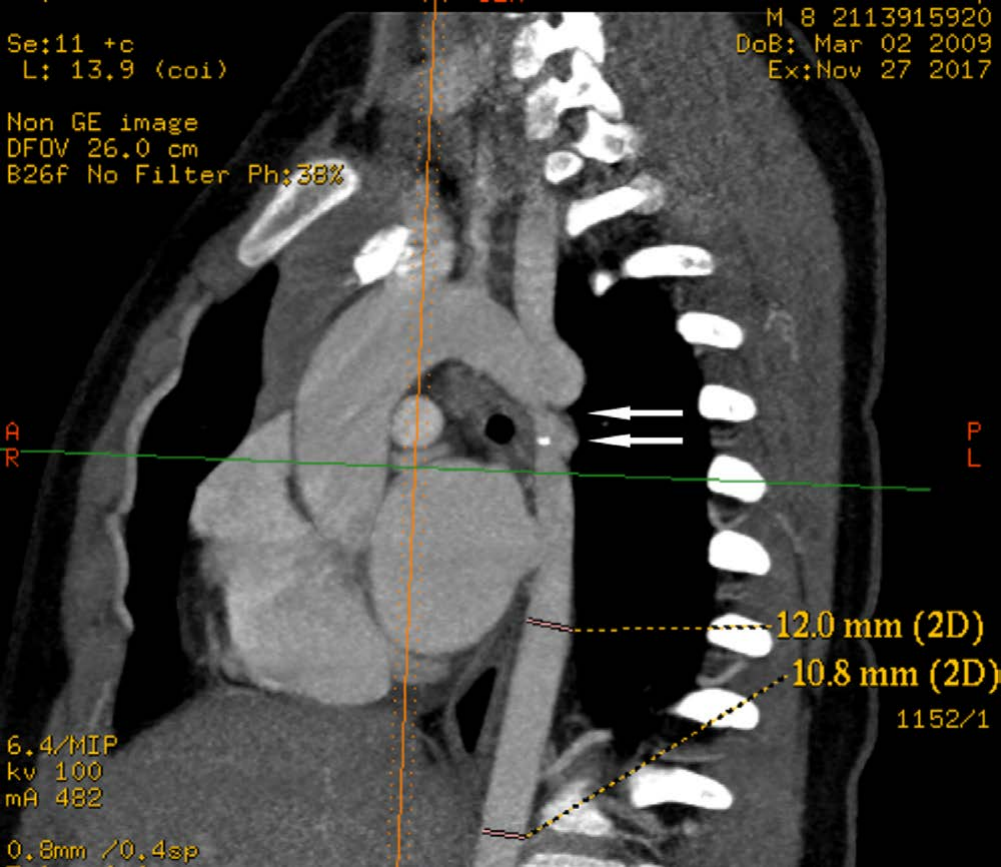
Cardiac CT angiography. The CT angiography suggested CoA (the upper arrow) with aneurysmal dilatation (the lower arrow). Two stenoses of the descending aorta can be seen, with a width of 3 to 4 mm at the narrowest point. CoA = coarctation of the aorta, CT = computed tomography.
